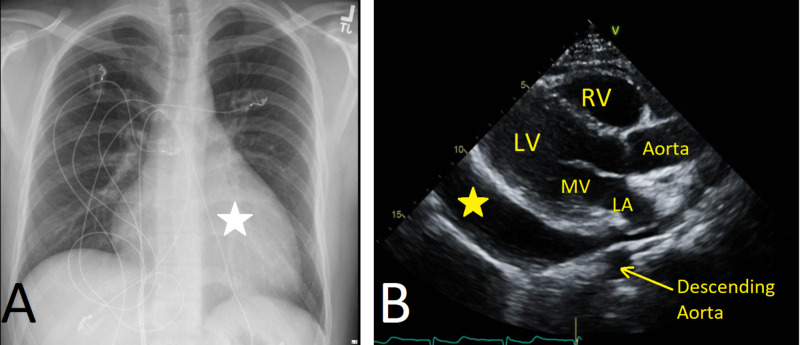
Imaging performed at the time of presentation. (A) Chest X-ray showing enlargement of the cardiac silhouette (white star). (B) Transthoracic echocardiography: parasternal long axis view showing pericardial effusion (yellow star). LV: left ventricle; MV: mitral valve; LA: left atrium; RV: right ventricle.
